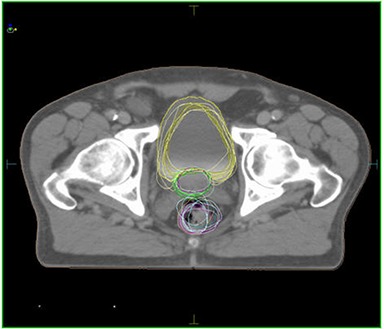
kvCT superimposed with kvCBCT contours showing variation in bladder and rectal volumes for a patient over a 9‐week period.
